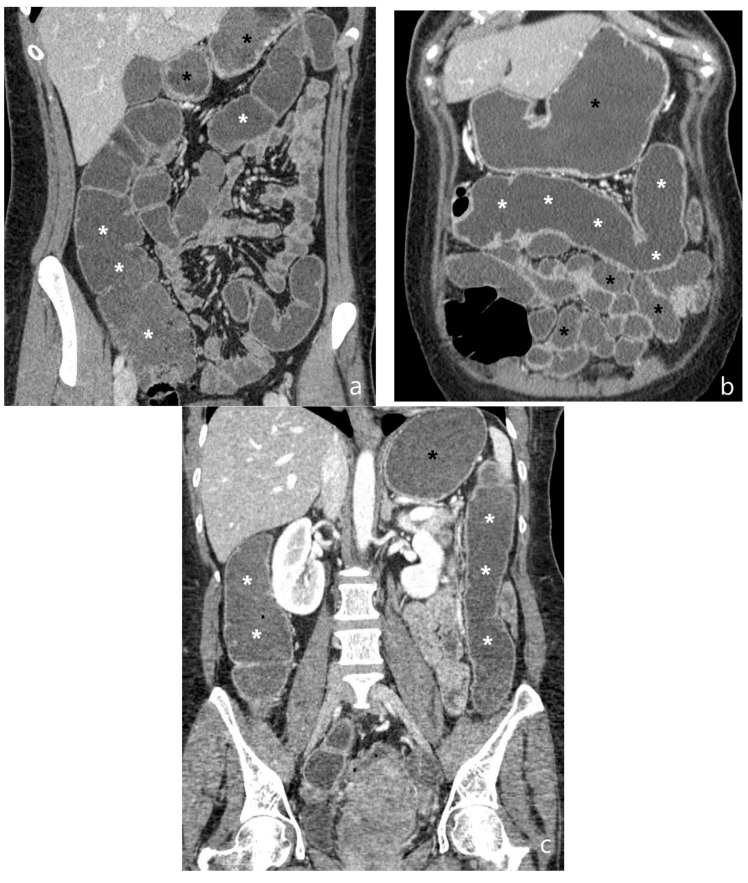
Hydro-CT. Coronal (a–c) images show good distension of the colon obtained by both endorectal (white asterisks) and oral (black asterisks) administration of water and hypodense contrast medium (polyethylene glycol solution). Original figures by LMM and LL.
